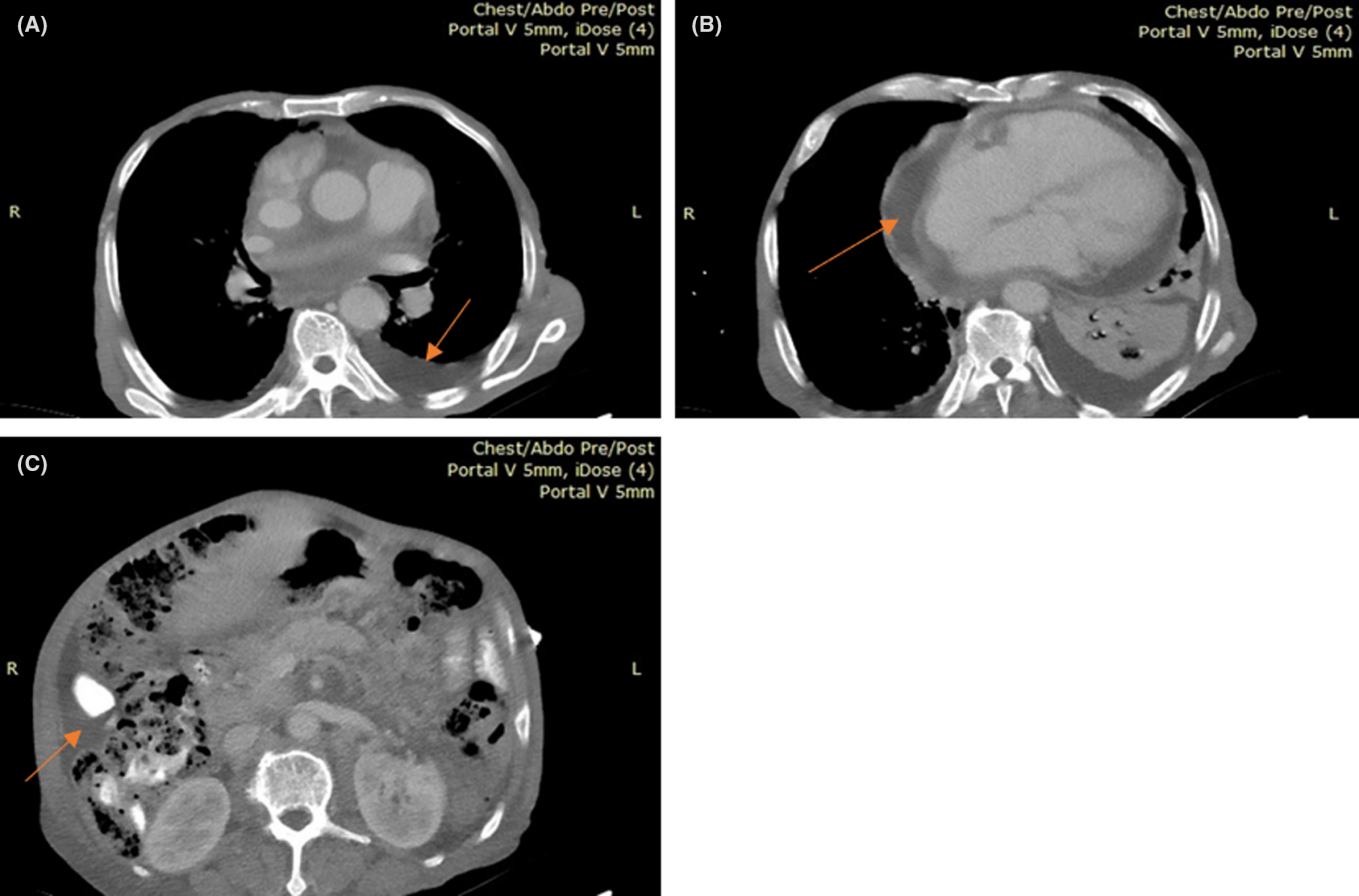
Chest/abdominal CT scan images showing pleural effusion (A), pericardial effusion (B), and ascites (C) pointed with the arrows.
